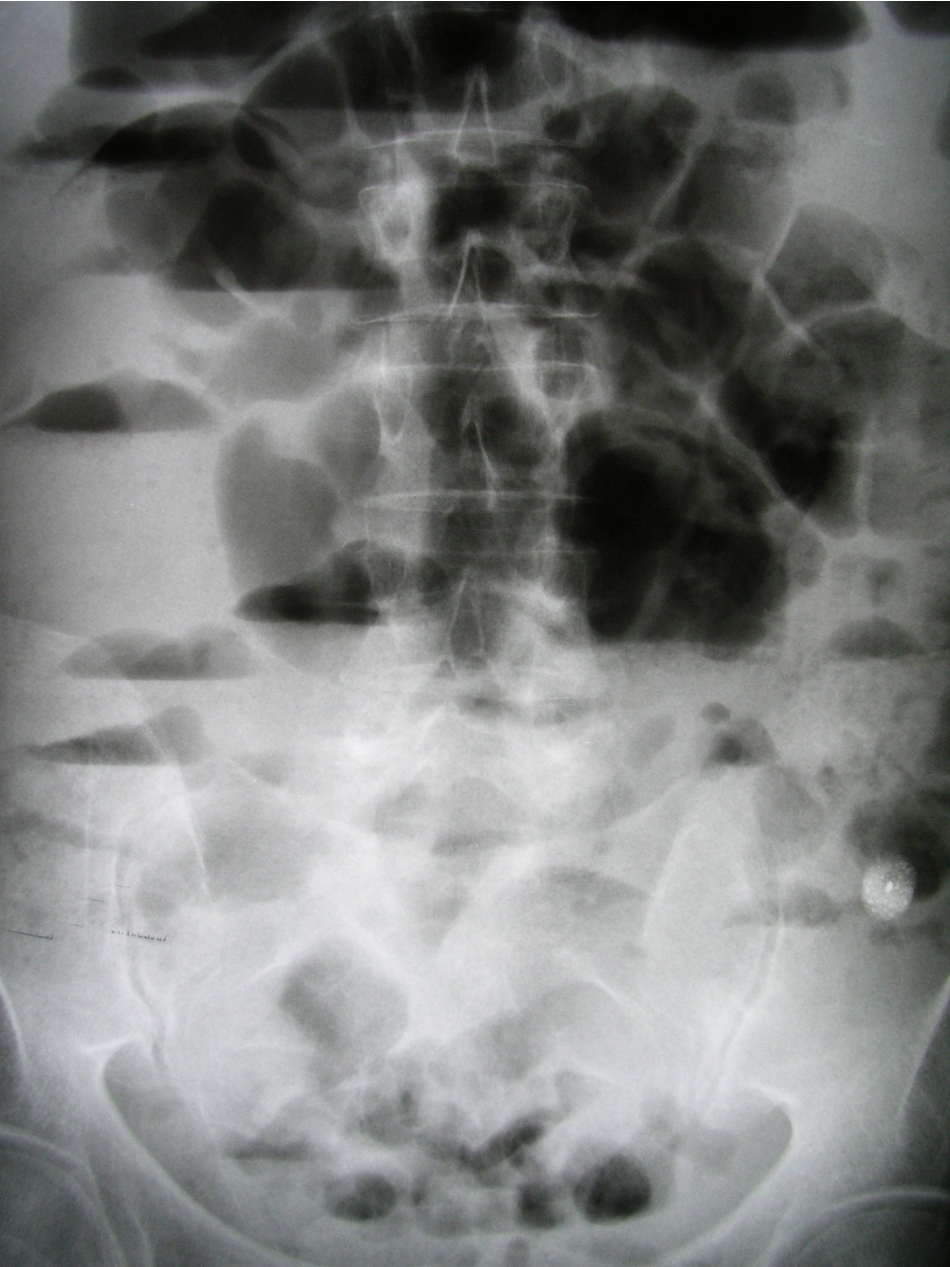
X-ray abdominal film on admission, showing distended small bowel loops and gas-fluid levels.
